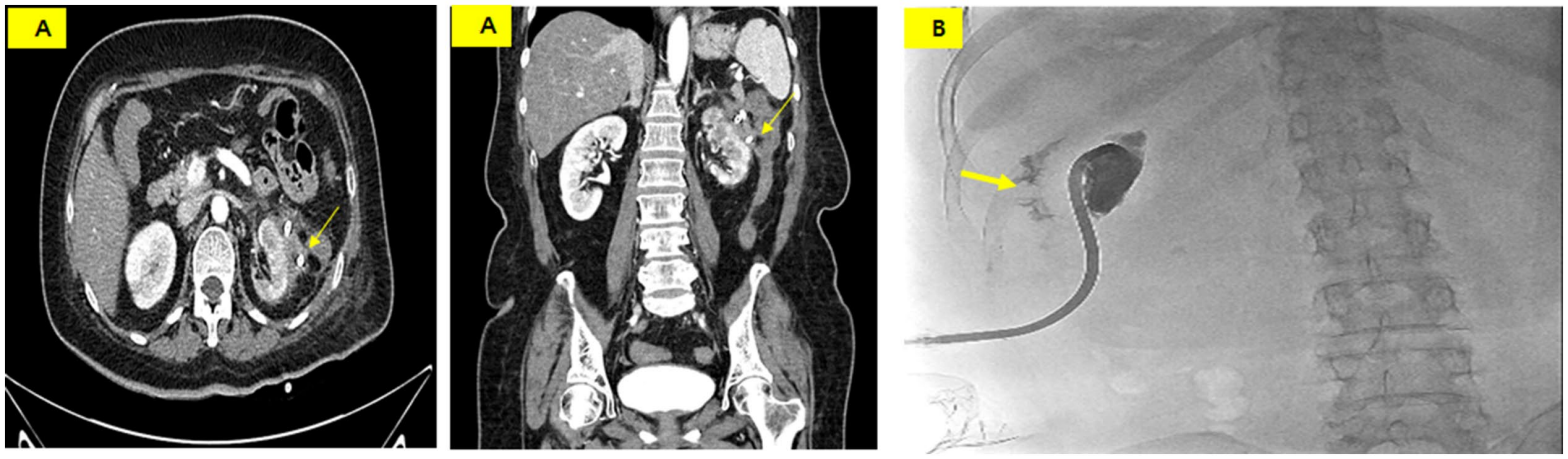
(A) CT scan on hospital day 60 showing a marked reduction of the abscess and a suspicious fistula or adhesion between the perinephric abscess and the descending colon (thin arrow). (B) Tubogram showing residual contrast leakage into the colon (thick arrow) but near resolution of the fistula tract.
